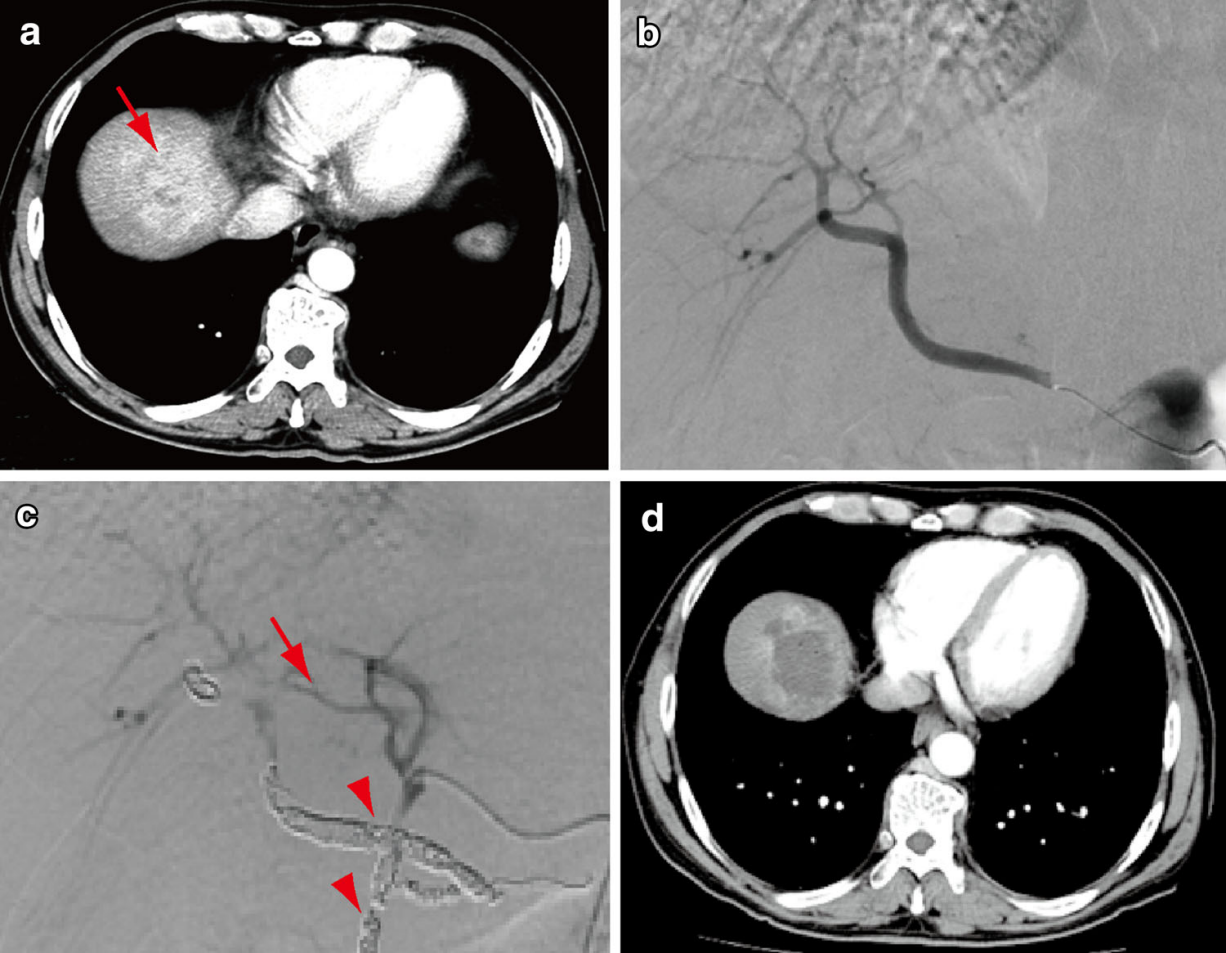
CT and angiography findings for case 2. a A CT image in the arterial-dominant phase demonstrates HCC (*arrow*) of approximately 50 mm in diameter in segments VII and VIII. b Supra mesenteric arteriography reveals the RHA branches from the SMA. c Celiac arteriography after occlusion of the GDA and replaced RHA using coils (*arrowheads*) shows communicating vessel (*arrow*) between the LHA and RHA. d A CT image in the arterial-dominant phase after port-catheter system implantation reveals a marked decrease in vascularity of the tumor
